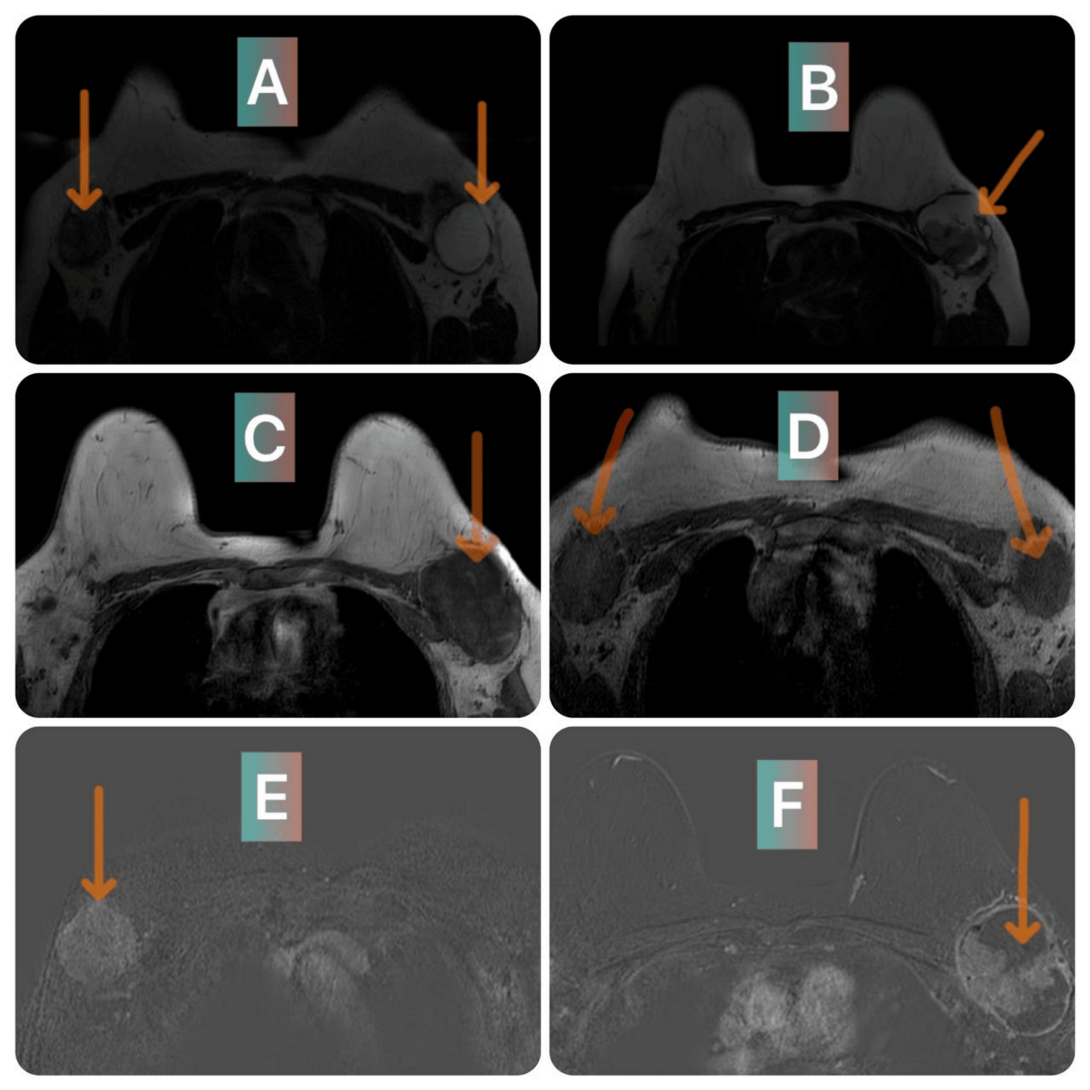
Breast MRI. T2-weighted (A and B) and T1-weighted (C and D) images with subtraction sequence (E) showing two masses in the axillary extensions, with irregular contours, containing a few liquid areas of necrosis, intermediate T2 heterogeneous signal, T1 hyposignal, restrictive to diffusion, and enhanced after injection of gadolinium (F).
